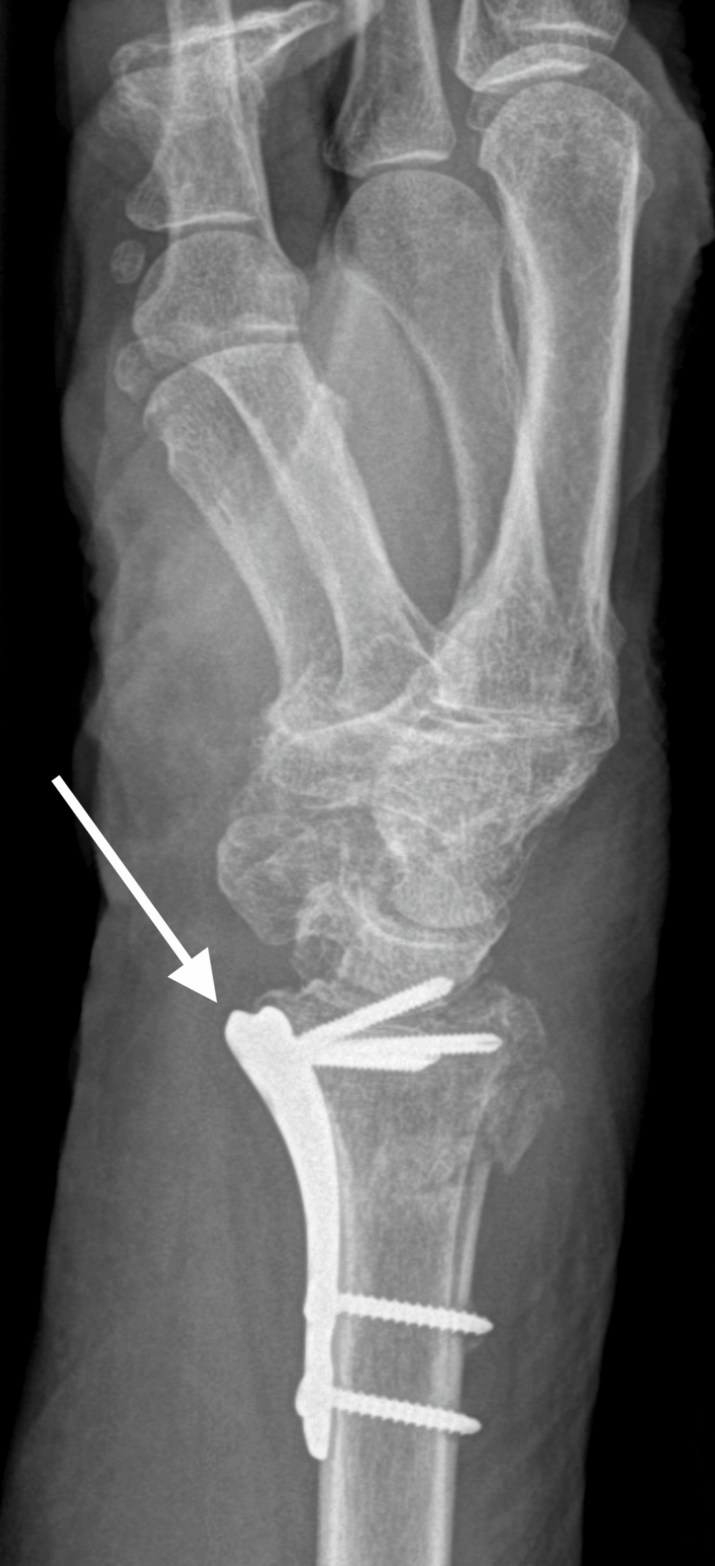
X-ray of the initial fracture at 14 months follow-up, lateral view, showing ongoing healing with encouraging signs of bridging callus formation
